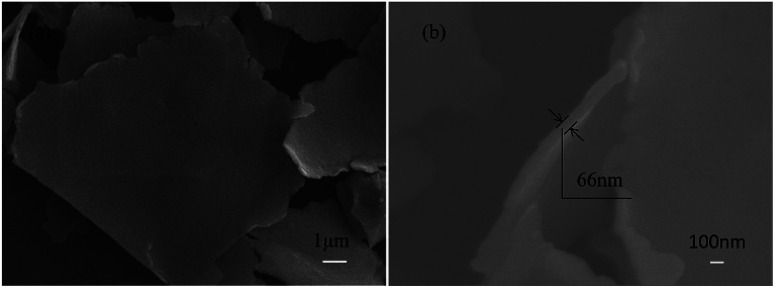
SEM images of the as-prepared silver flakes that were ultrasonically treated for 60 minutes: (a) surface appearance and (b) cross-section.
